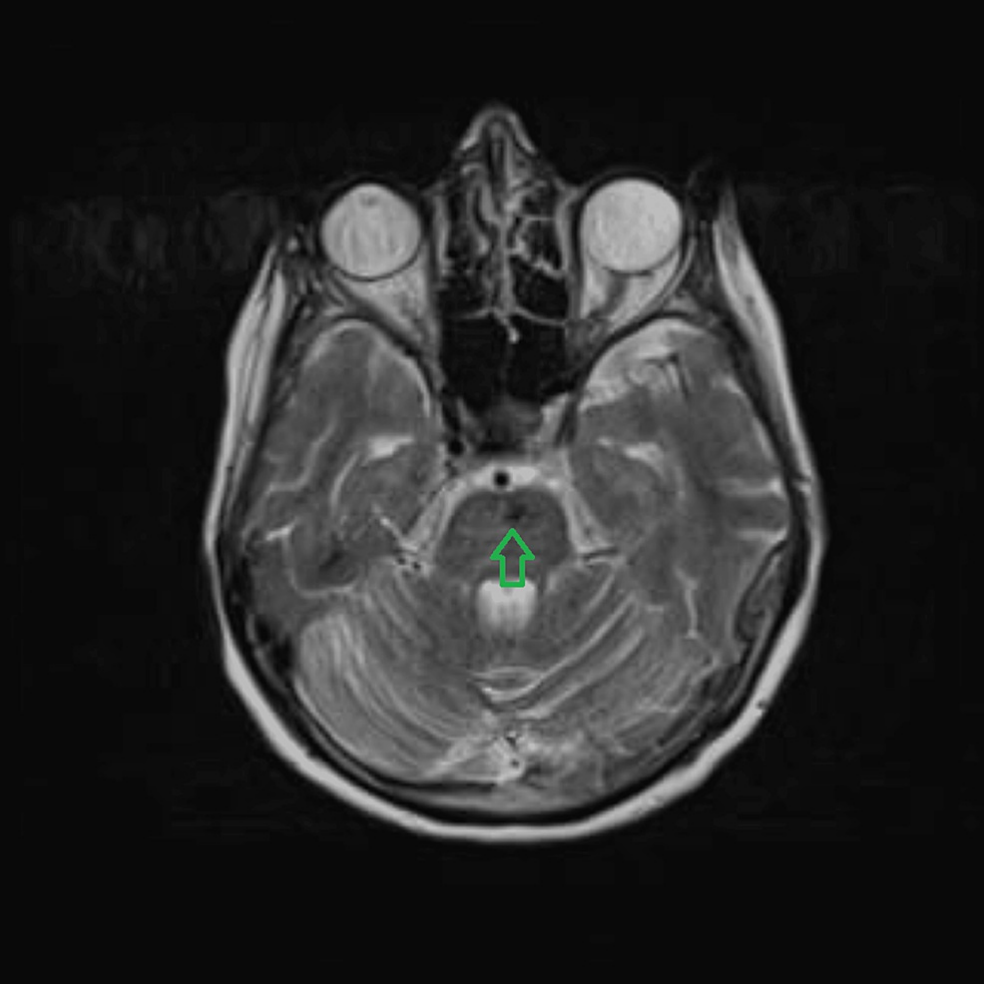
MRI brain showing pontine haemorrhage corresponding to CT head pontine haemorrhage CT: computed tomography; MRI: magnetic resonance imaging
